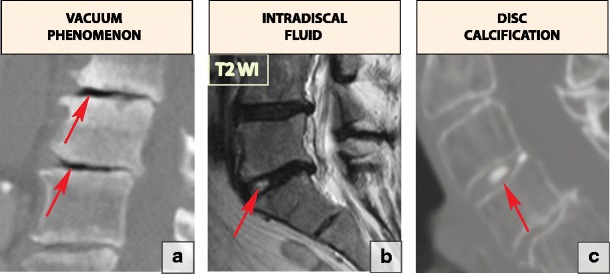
Signs of intervertebral disc degeneration: (a). The vacuum phenomenon. This sagittal CT reformatting image shows the foci of air within the L2–L3 and L3–L4 discs (arrows). (b) Intradiscal fluid accumulation (arrow). (c) A sagittal reformatting CT image at the level of C3–C4 shows disc calcification (arrow)
